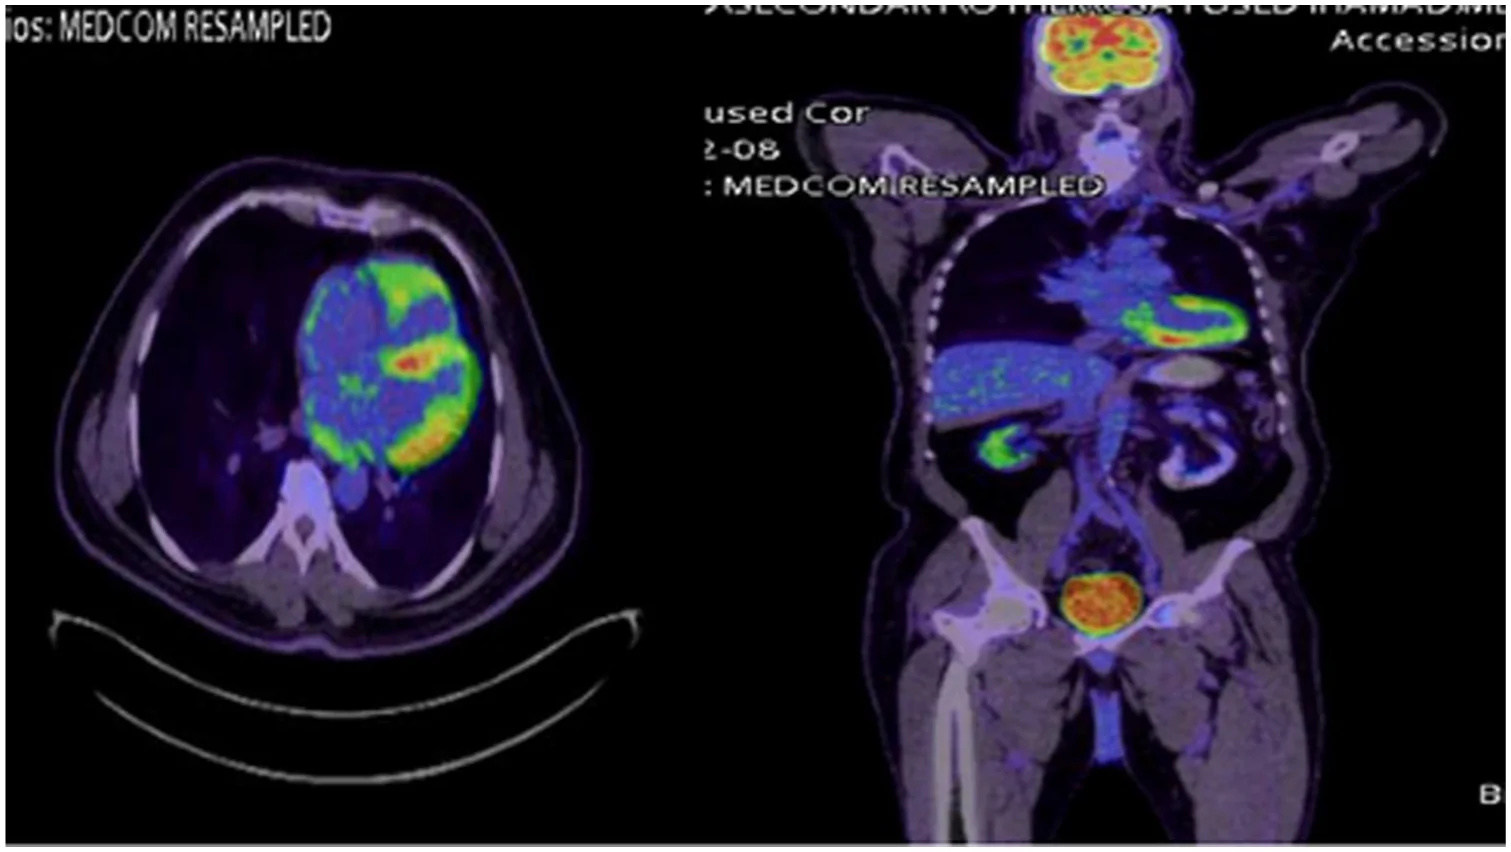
Fused PET/CT images of the thorax and whole body. Fused axial (left) and coronal (right) PET/CT images demonstrating a hypermetabolic left atrial mass with high FDG uptake. No evidence of distant metastases was observed on whole-body screening.
